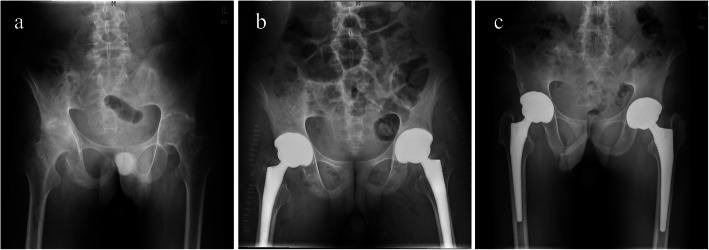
Case presentation of synchronous cementless bilateral total hip arthroplasty for osseous ankylosed hips with ankylosing spondylitis a: A man was diagnosed with ankylosing spondylitis and showed bilateral osseous ankylosed hips preoperatively. b: The radiograph of the pelvis after surgery immediately showed similar inclination of cup and femoral off-set as well as small leg-length discrepancy c: The radiograph of the pelvis at 100-month follow-up showed no aseptic loosening and migration of the component
